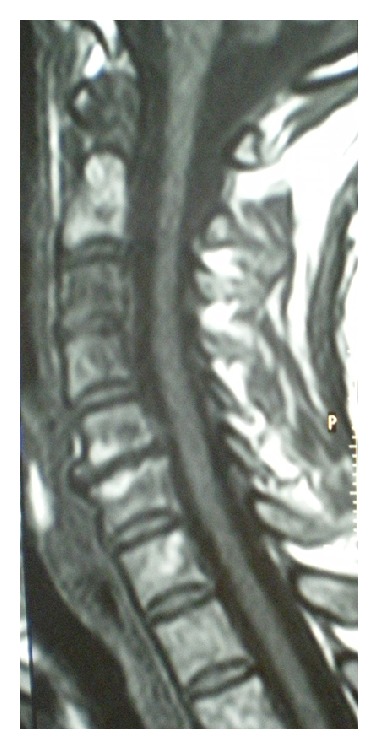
MRI sagittal view of the cervical spine showing a C5-C6 degenerative disc disease and generalized spondylosis of the entire cervical spine.
